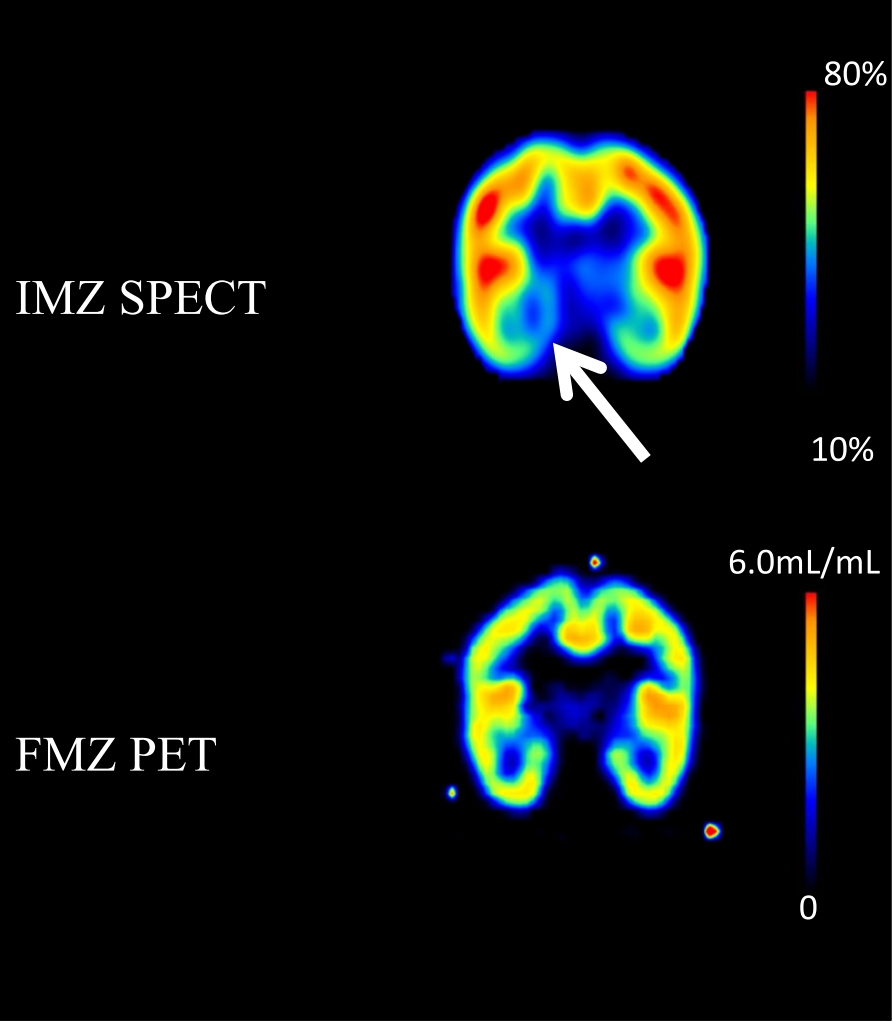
Case 3 (Patient No. 5): The patient was a 21-year-old male who was diagnosed as having right frontal brain contusion at the time of injury and complained of mental fatigue after the injury. The uptake level of IMZ SPECT was low at the right basal-medial temporal lobe. But the low BP_ND_ was not observed by FMZ PET
